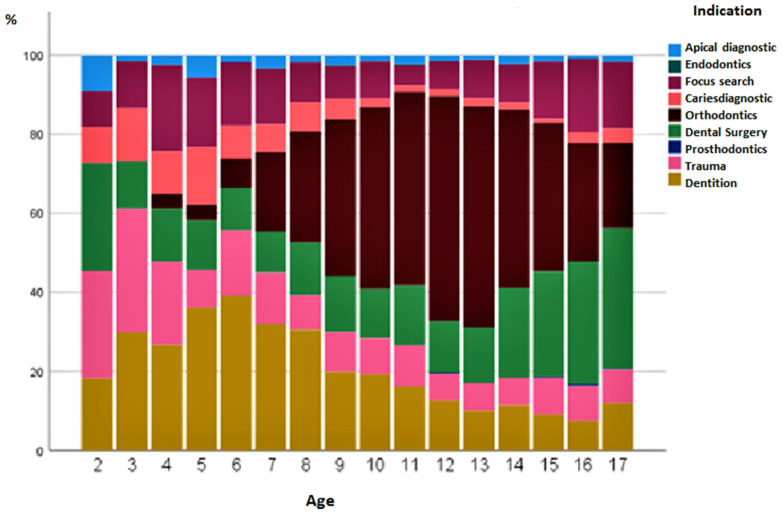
Distribution of dental indications for radiography from 1 to 18 years of age.
